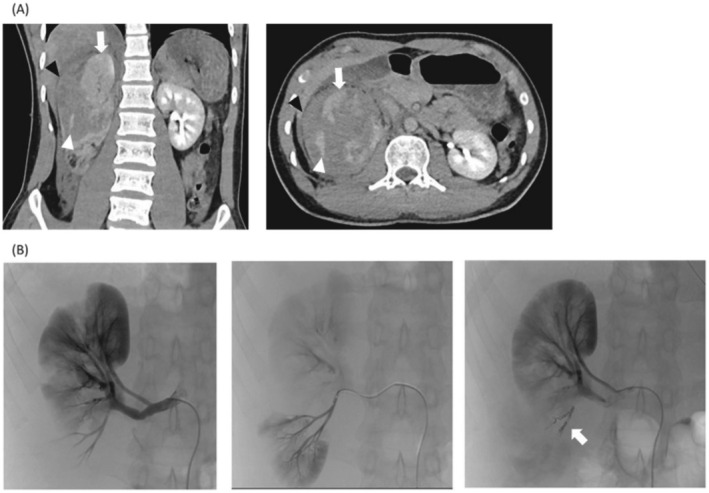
(A) Contrast‐enhanced computed tomography on the day of injury demonstrating a right renal parenchymal hematoma with urinary extravasation extending into the retroperitoneal space. White arrow: Renal parenchyma, Black arrow head: Hematoma, White arrow head: Urinary extravasation. (B) Angiographic findings during TAE demonstrating separation of the lower pole from the main renal parenchyma; coil embolization of the lower pole branch (arrow) was performed with adjunctive use of a small amount of gelatin sponge slurry.
